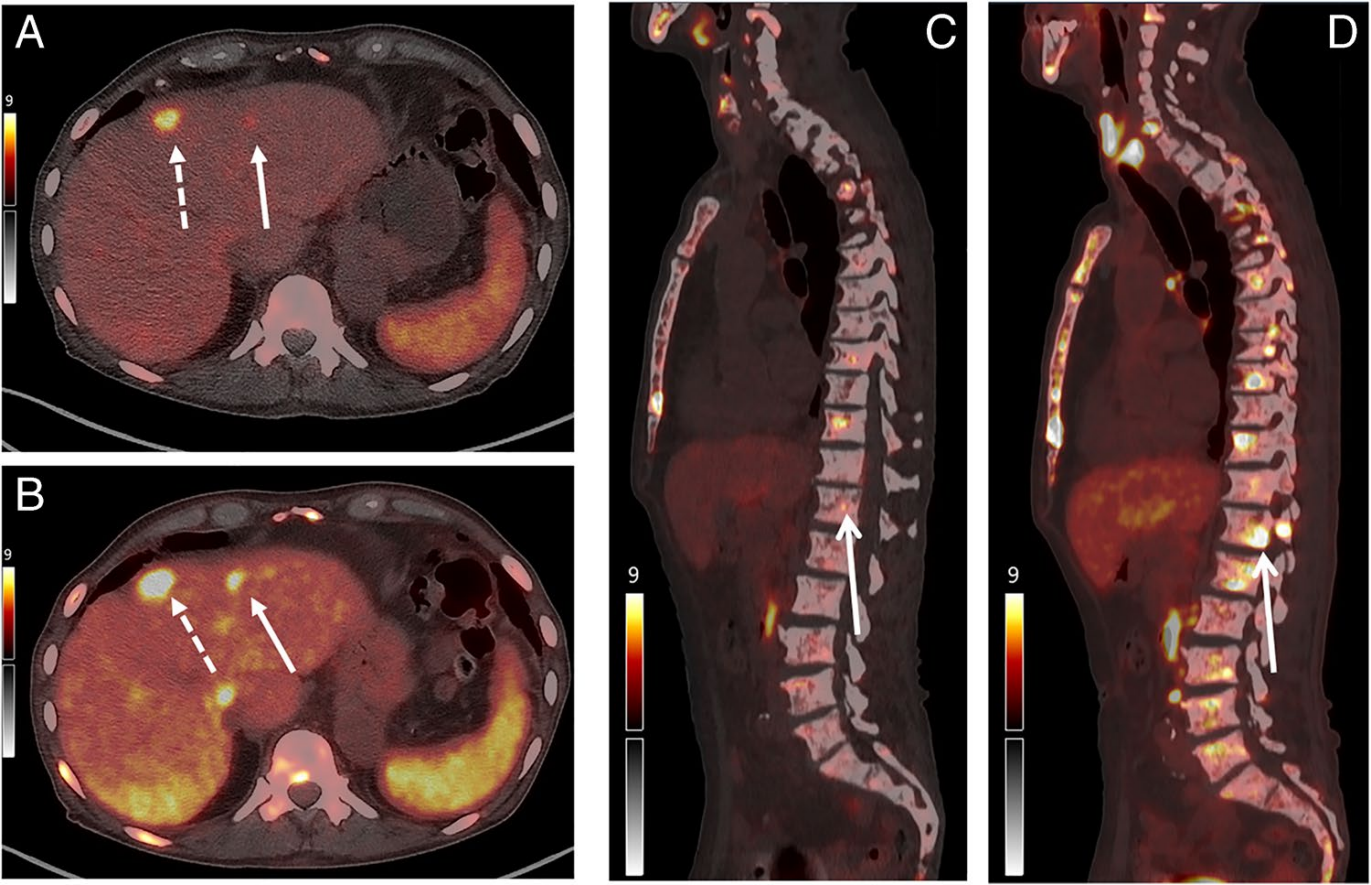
Example images for a patient with mismatch lesions. A and C show the [^68^ Ga]Ga-PSMA-11 PET/CT performed at 1 h p.i. and B and D the additional PET/CT 1 h following additional administration of FDG. Although the lesion shown by the broken arrow demonstrates increased uptake at second imaging, the hepatic lesion shown by the solid white arrow in A and the example bone lesion in C show intense FDG uptake at the second PET/CT (B and D) which is indicative of low PSMA expression and high FDG avidity
